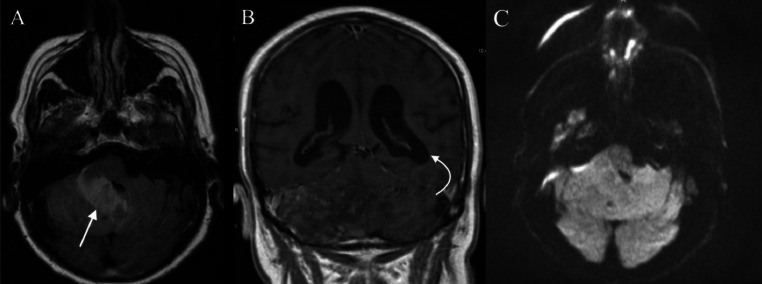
Fluid attenuated inversion recovery (FLAIR) MRI brain from initial hospitalization showed an area of hyperintensity within the right cerebellar hemisphere (straight arrow) on axial imaging (A), causing mass effect on the fourth ventricle. Associated hydrocephalus (curved arrow) was appreciated on coronal imaging (B). Diffusion-weighted imaging (DWI) was negative for diffusion restriction (C).
